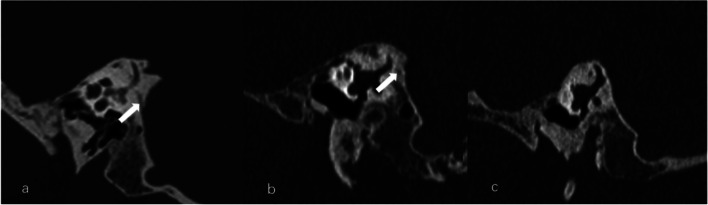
The visibility of vestibular aqueduct (VA) grading on the Pöschl planes on temporal bone CT. a Grade 0 with a continuous VA (arrow). b Grade I with a discontinuous VA (arrow). c Grade II with a complete ossification of VA
